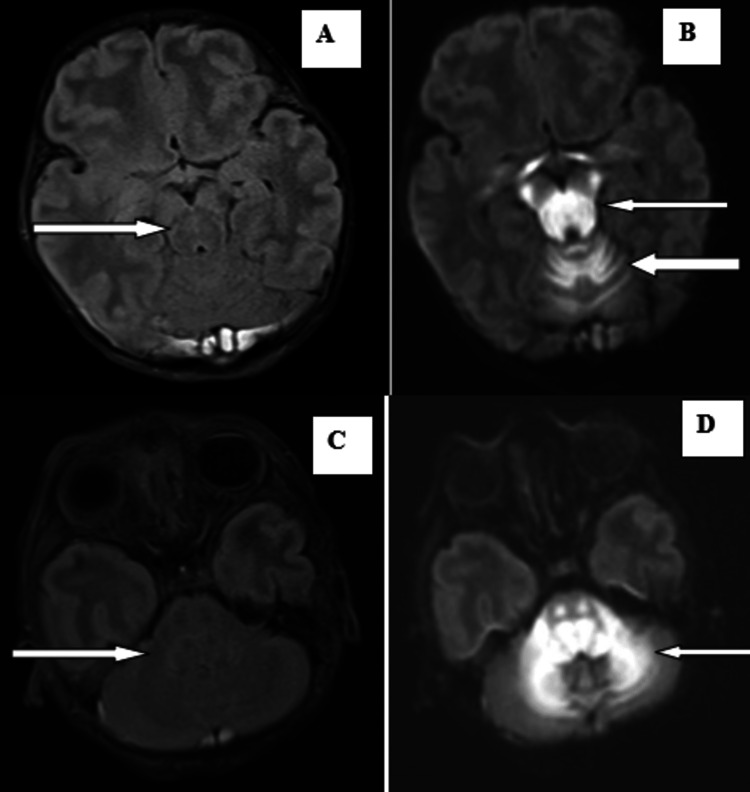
Subtle FLAIR hyperintense signals are noted in the dorsal aspect of the midbrain, pons, medulla, vermis, and cerebellar white matter (A, C). Diffusion restriction is noted in the dorsal aspect of the midbrain, pons, medulla, vermis, and cerebellar white matter (B, D). FLAIR: fluid-attenuated inversion recovery
